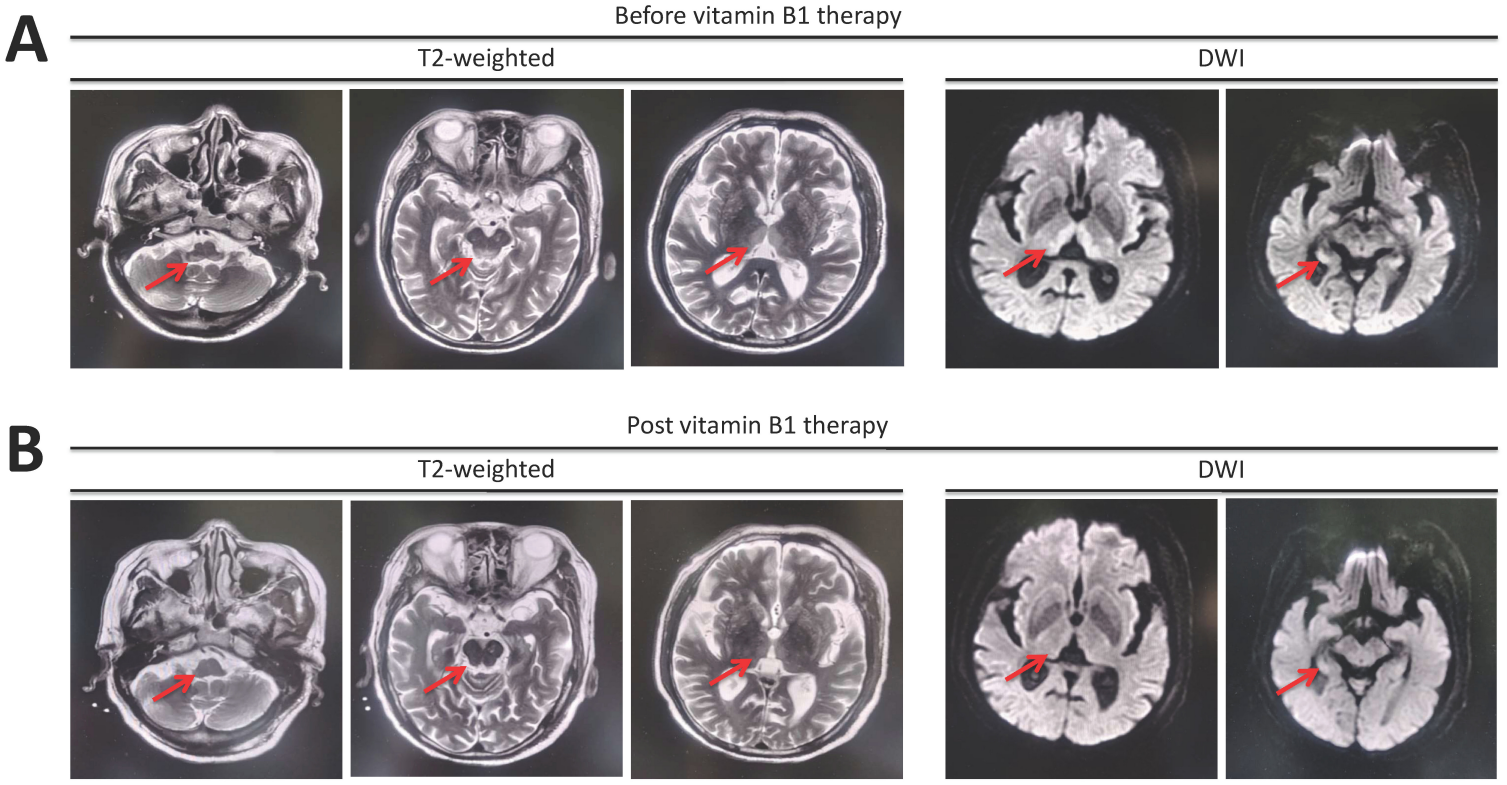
Magnetic resonance imaging manifestation reveals (A) symmetric hyperintense signals on T2-weighted images in the bilateral inferior cerebellar peduncle, dorsal pons, and medial thalami as well as increased signal intensities on diffusion-weighted images (DWI) in bilateral thalami and hypothalamus. (B) The lesions were reversible after 12 days of intravenous vitamin B1 supplementation.
